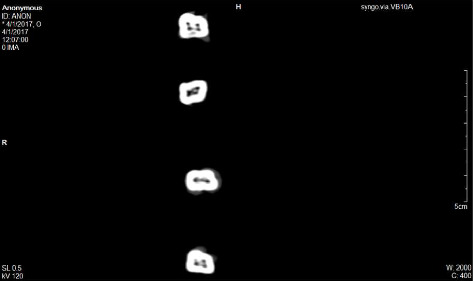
An image of a CT slice at level of floor of pulp chamber showing number of root canal orifices in each root of a tooth.
